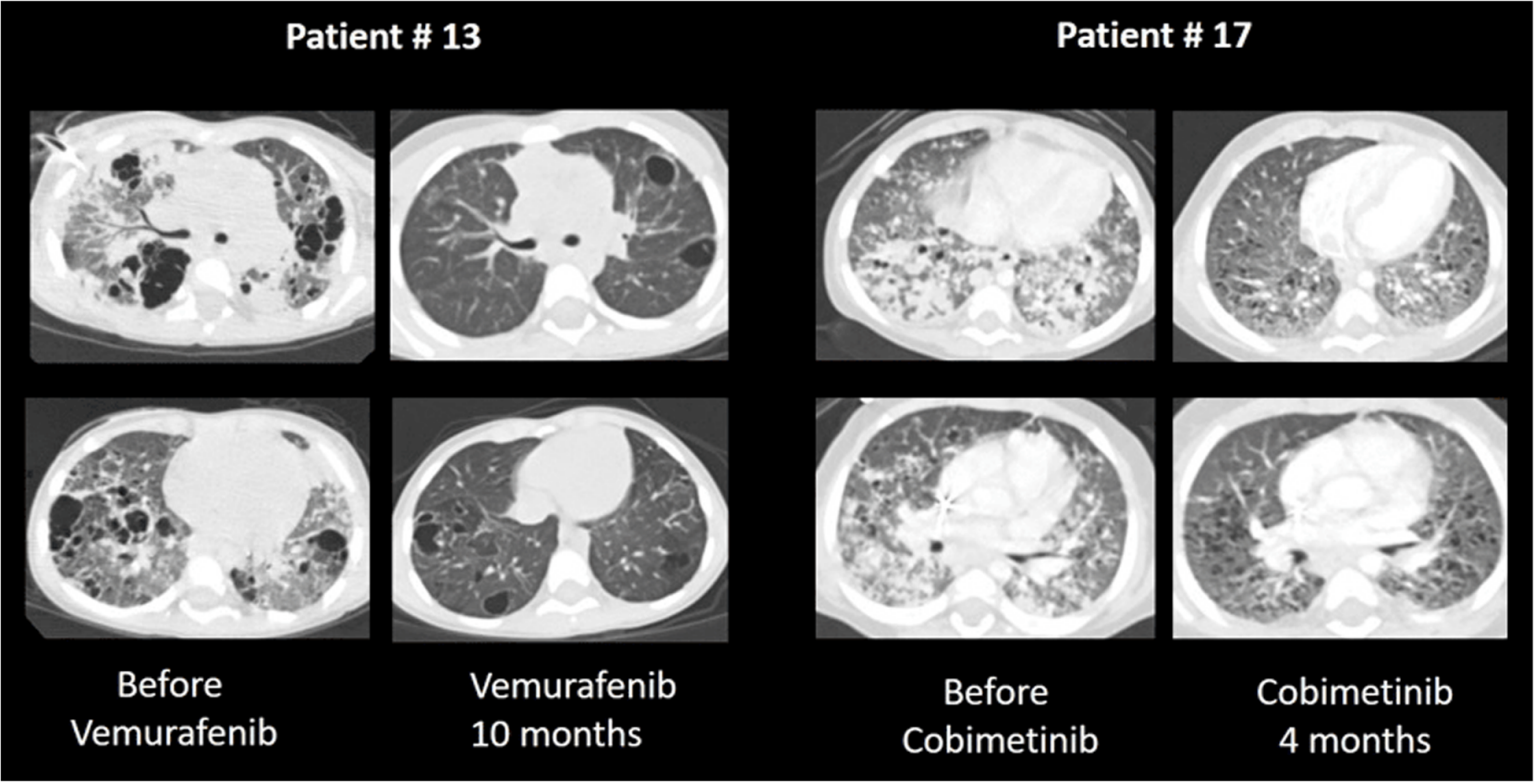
Chest CT scan images (lung window) before and after initiation of *target therapy.* Images show lungs before and after the initiation of a BRAF-kinase inhibitor (Vemurafenib) and a MEK inhibitor (Cobimetinib)
